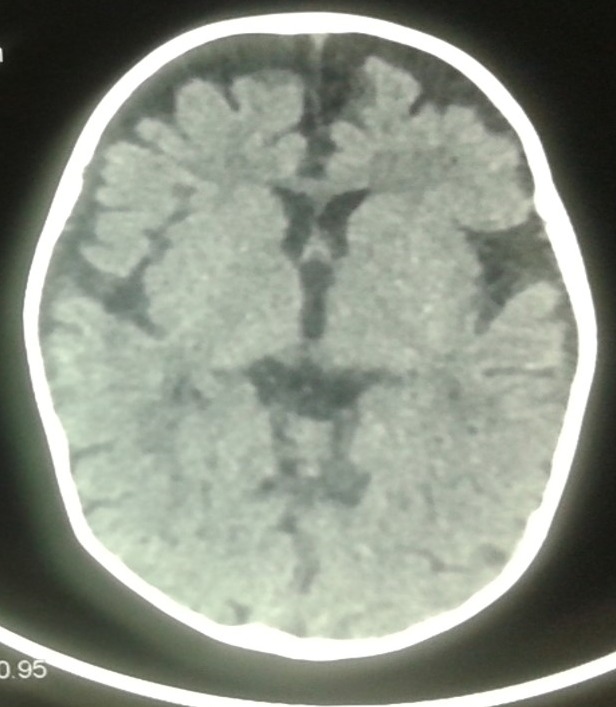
Brain CT revealed cerebral atrophy and delayed myelination
